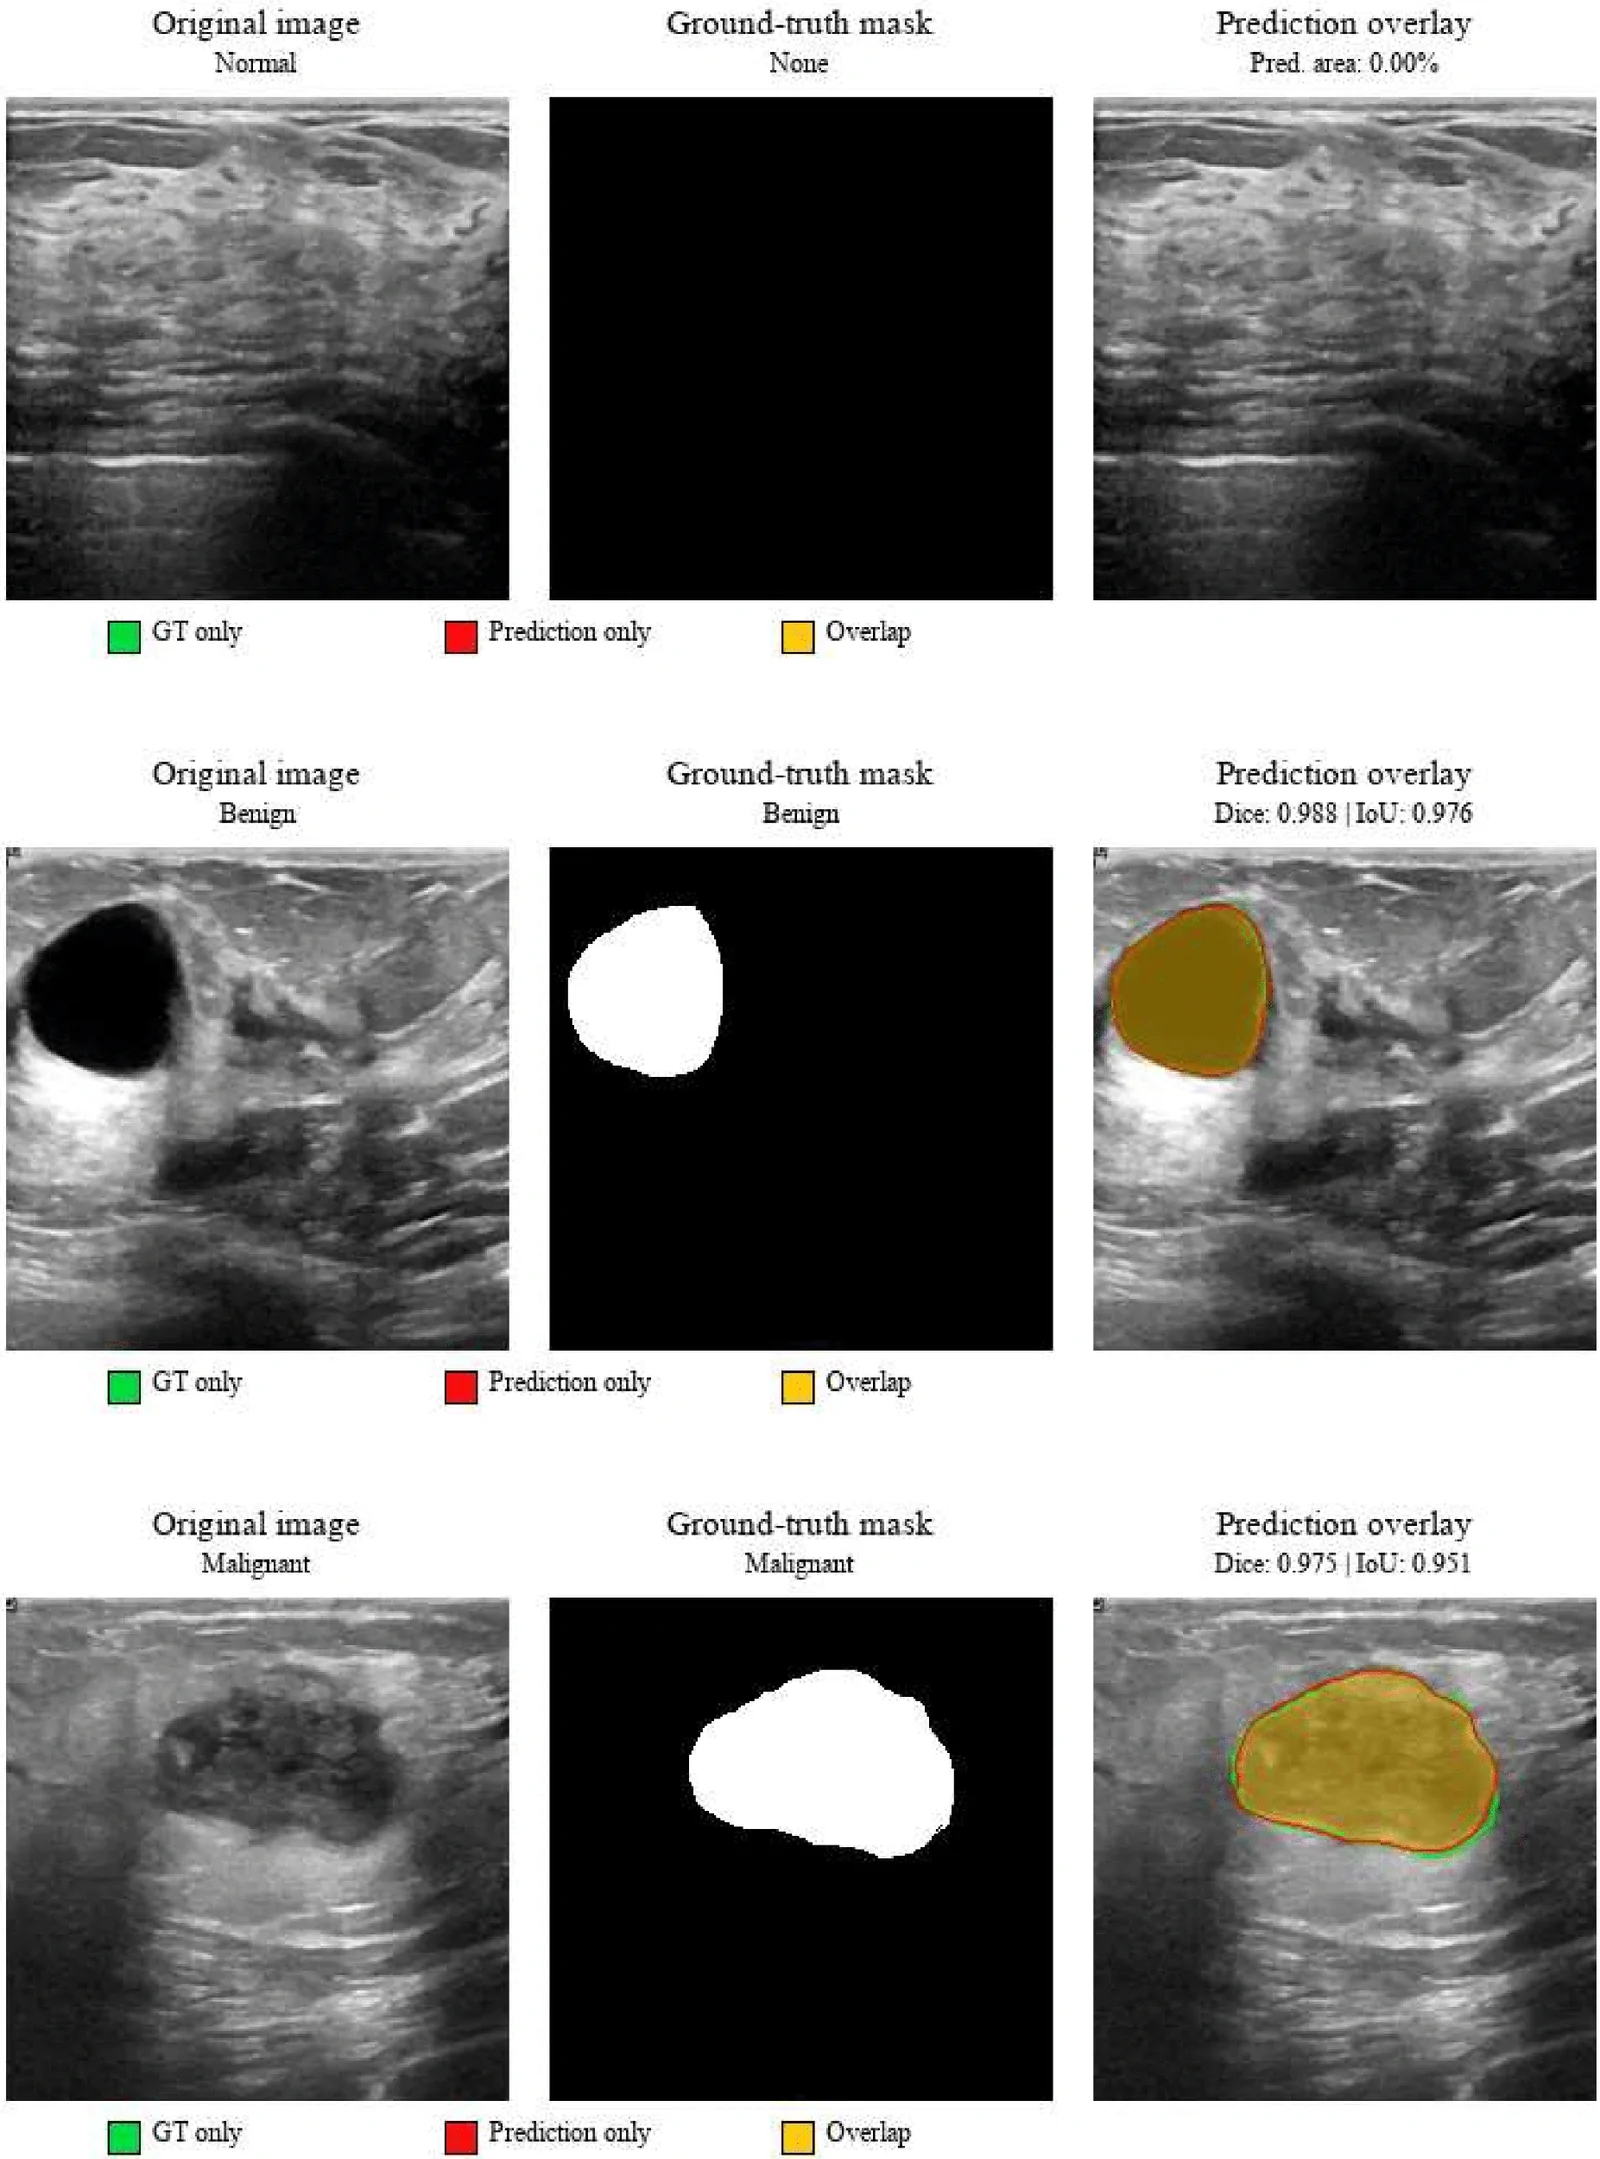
Representative segmentation examples generated using the trained FET UNet models. For each class, the original ultrasound image, ground-truth mask, and prediction overlay are shown. In the overlay, yellow represents correctly segmented overlap, green represents missed ground-truth regions, and red represents extra predicted regions.
